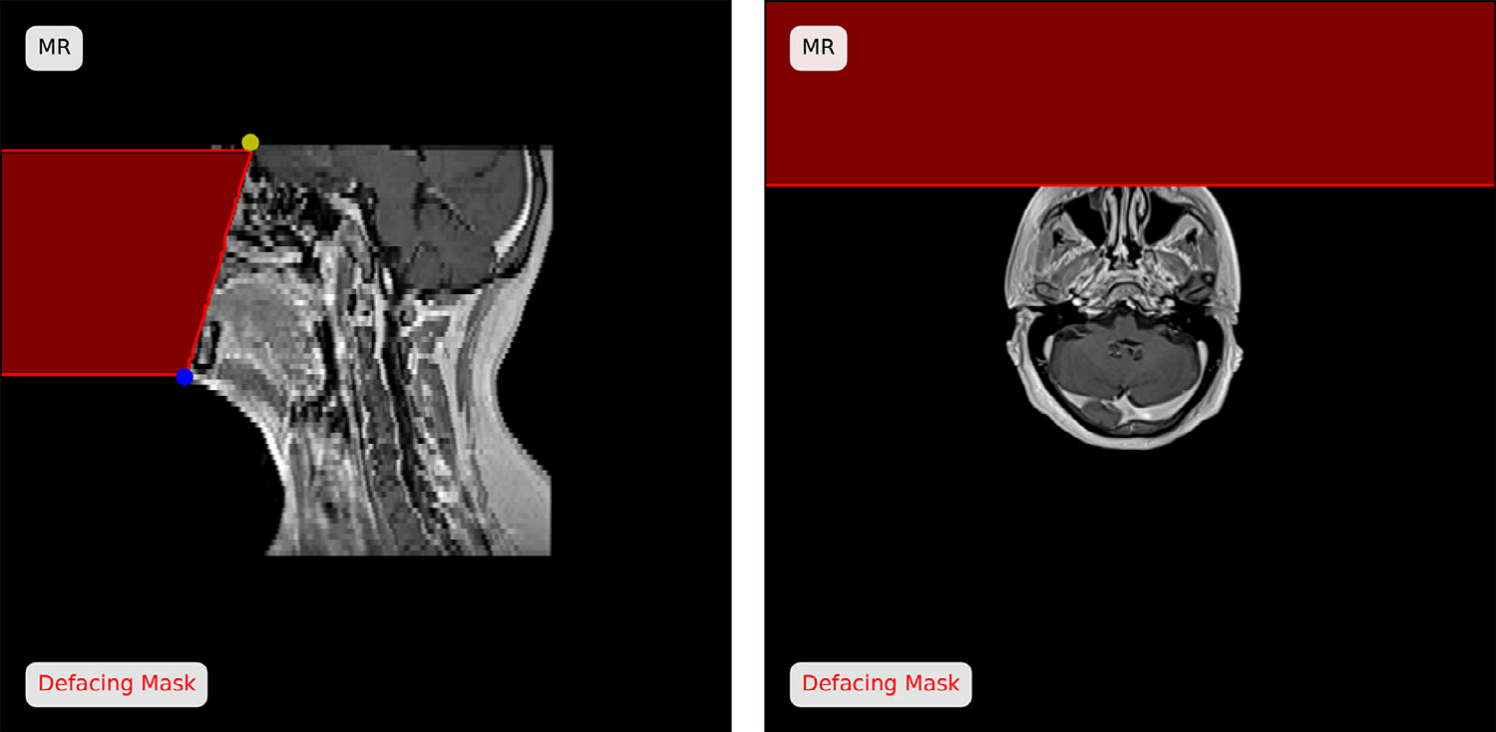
Example of the automatic defacing algorithm for the SynthRAD2025 dataset. The red area indicates the region overwritten with background values during the defacing. The yellow and blue markers indicate the points defining the defacing mask and were derived from the auto‐segmented brain (yellow) and mandible (blue) structures on the corresponding CT.
